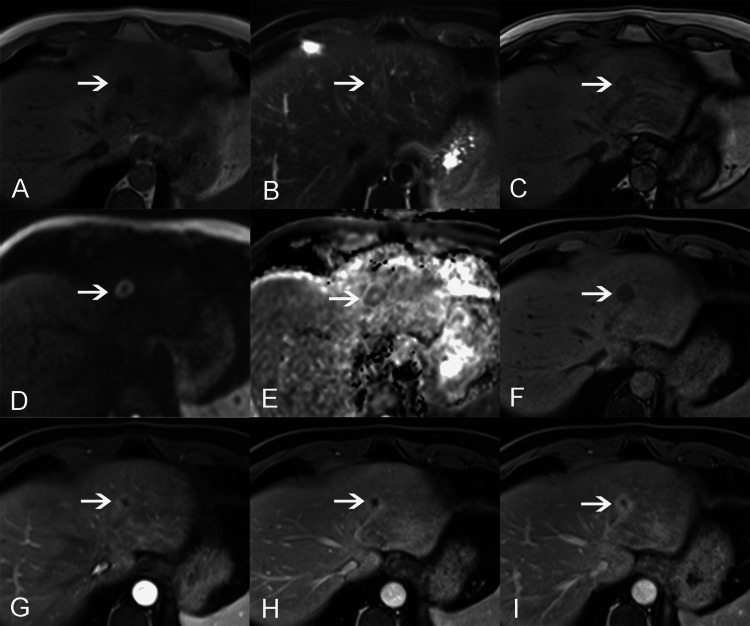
MRI findings. MRI revealed a round mass (arrows) with a clear edge in segment 2 of the liver. It showed low signal intensity on T1WI and high signal intensity on T2WI (A,F,B). The fat component was not demonstrated (C). DWI MRI showed a ring of high intensity (D). The rim of the lesion appeared slightly hypointense to the surrounding parenchyma on ADC maps (E). Gd-DTPA dynamic enhanced scanning showed a prolonged “ring enhancement” pattern (G-I).
